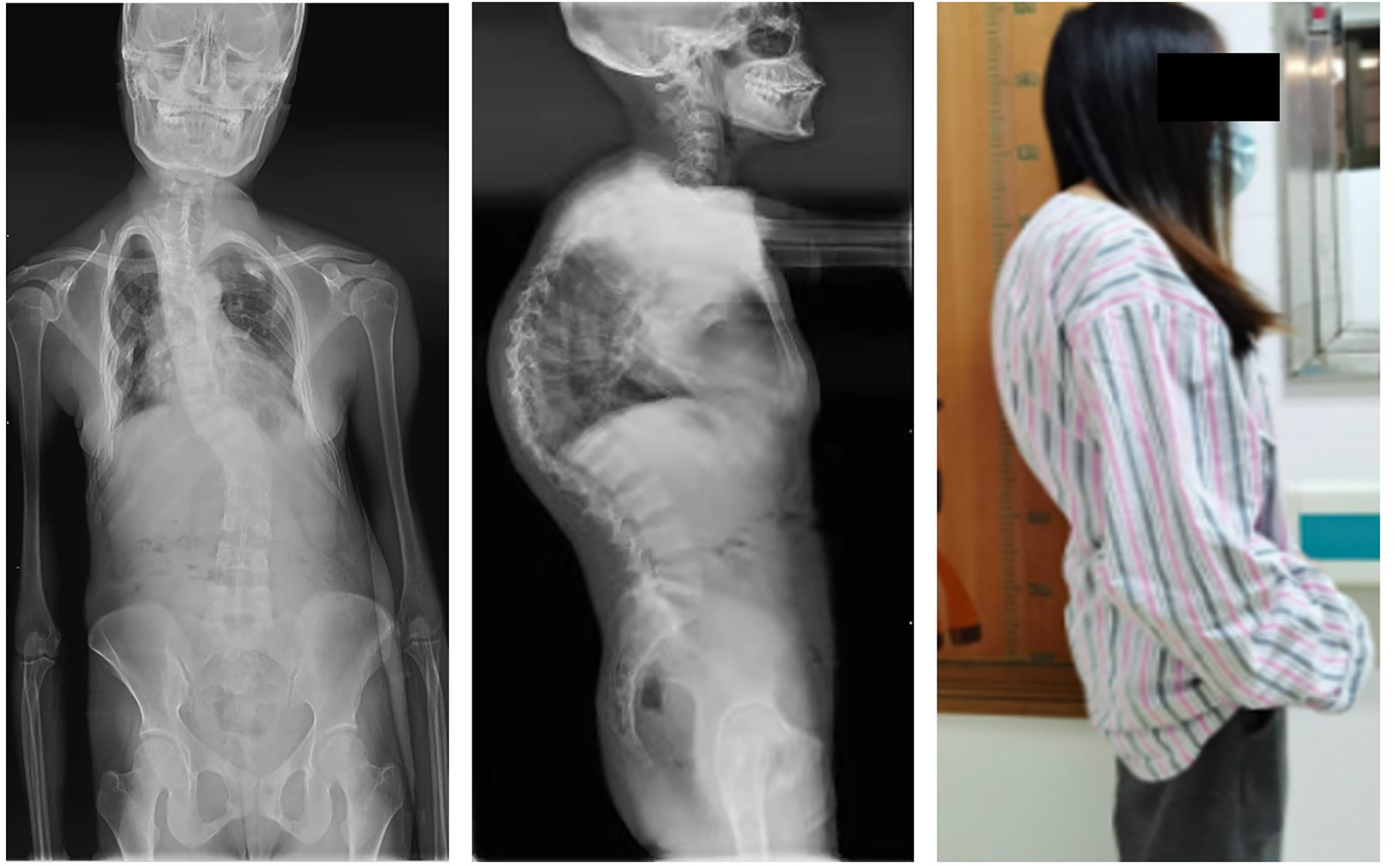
Whole-spine splicing X-ray anterior and lateral views. At the onset of the disease, the adefovir patient obvious severe scoliosis: rightward bending deformity.
